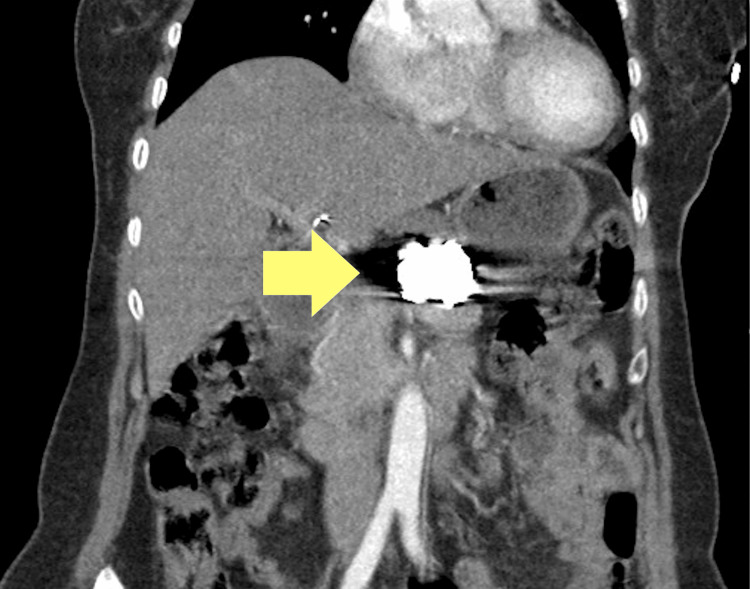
Follow-up CT angiography one year post embolisation showing complete splenic artery aneurysm sealing.
